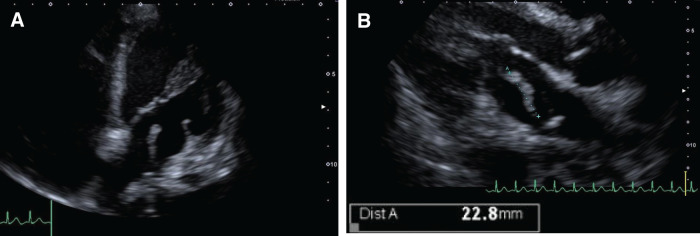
Transthoracic echocardiography findings. (A) A mobile mass is attached to the posterior wall of the left atrium. (B) The mass has a length of approximately 22.8 mm.
